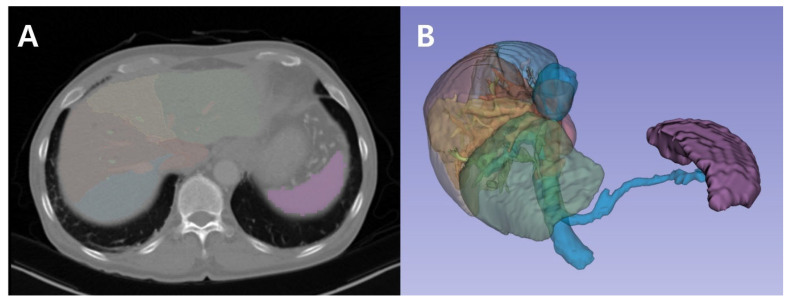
Spleen volumetry using 3D Slicer’s Total Segmentator. (A). Segmented spleen from abdominal CT scan. The spleen is highlighted in purple, with the liver faintly visible, to demonstrate their anatomical structures as viewed from the axial plane (horizontal cross-section). (B). 3D volumetric rendering of the spleen. The spleen is highlighted in purple, along with its associated vascular structures and connection to the liver.
